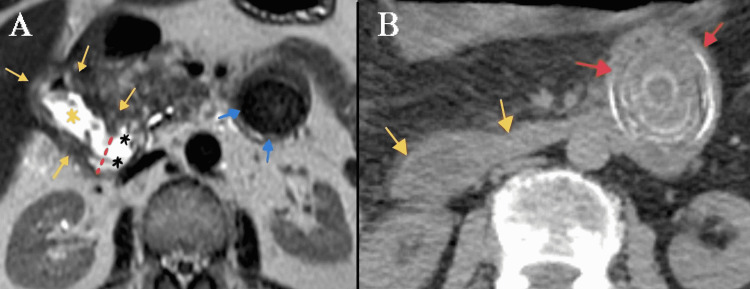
(A) Axial T2-weighted MRI section: Prominently thickened gallbladder wall (yellow arrows) and gallbladder lumen (yellow asterisk) are shown. Cholecystoduodenal fistula (red dashed line) connects gallbladder lumen to duodenal lumen (black asterisks). Giant gallbladder stone (blue arrows) in the proximal jejunum can also be seen; (B) Axial non-contrast CT image section: Duodenal segments (yellow arrows) distal to cholecystoduodenal fistula and giant gallbladder stone (red arrows) in the proximal jejunum are seen.
